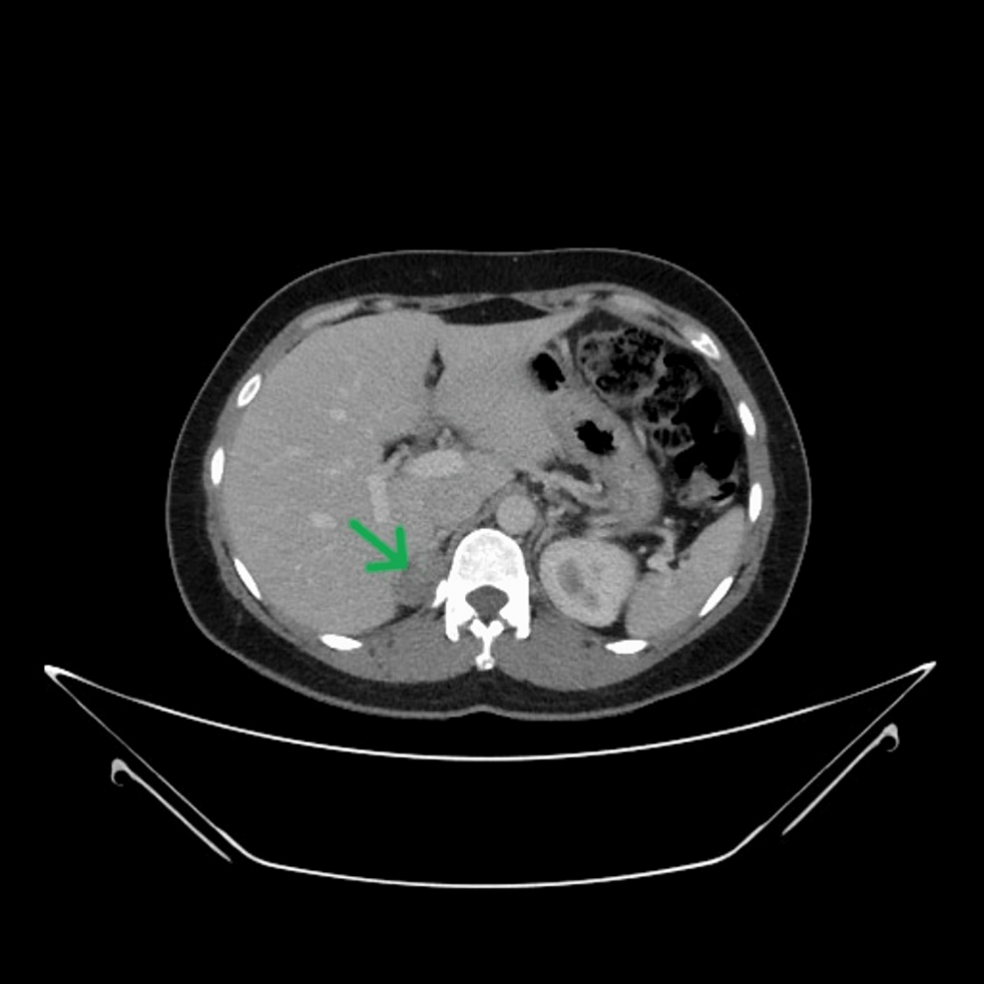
Computed tomography of the abdomen - adrenal adenoma pointed with arrow.
